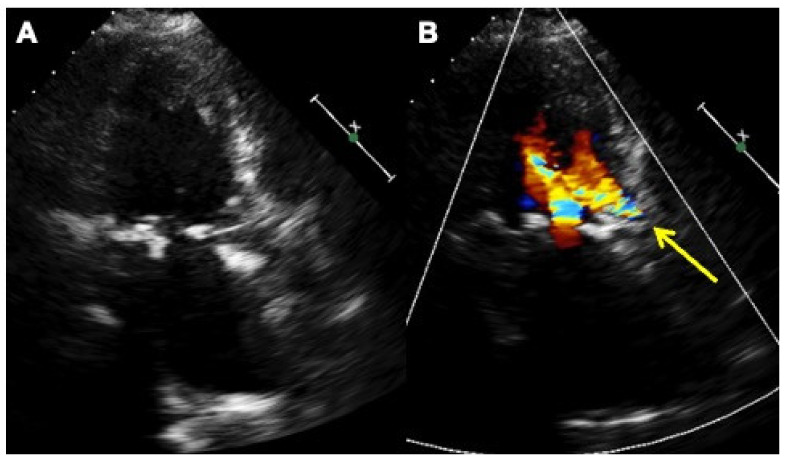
Two-dimensional transthoracic echocardiography color Doppler in two-chambers plane without (A) and with color Doppler (B) showing the self-expandable aortic biological valve in place, the paravalvular leak at the left coronary aortic cusp with the corresponding regurgitant jet (yellow arrow).
